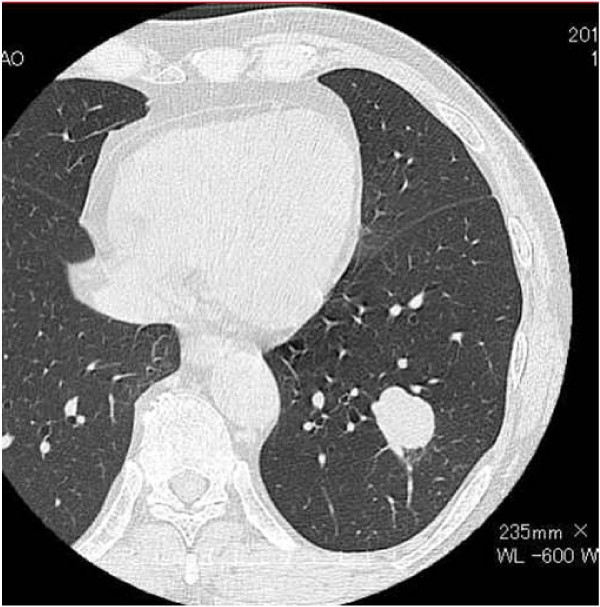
CT showed a mass shadow measuring 30 mm in diameter in S9.
